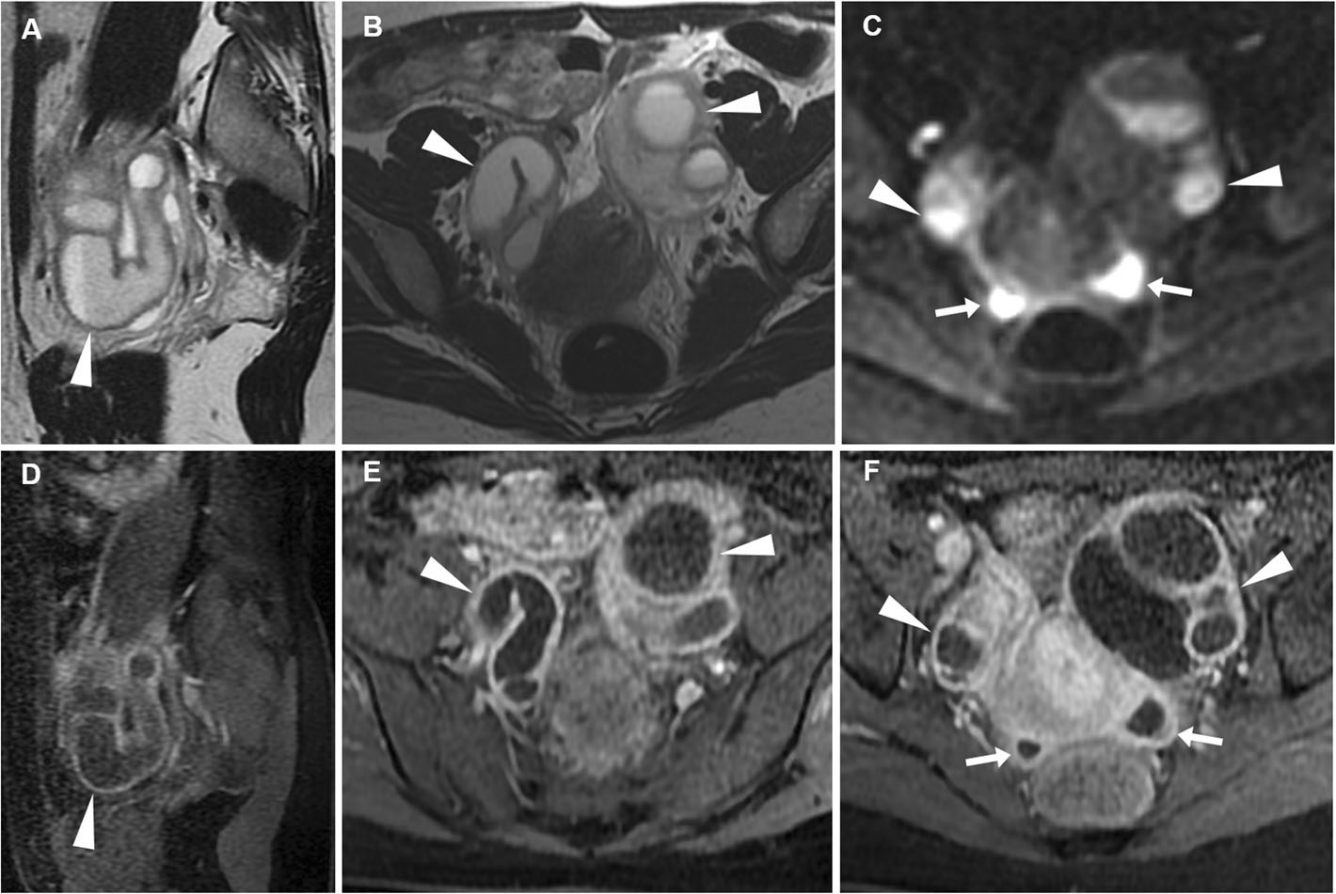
Acute pyosalpinx in the same patient as Fig. 11. Sagittal (a) and oblique-axial T2-weighted (b) images show distended fallopian tubes (arrowheads) with thickened walls and internal fluid-fluid levels. Axial DWI (*b* = 800 s/mm^2^) image (c) demonstrates high signal intensity indicating restricted diffusion of the tubal content (arrowheads) and of two fluid collections in the Douglas pouch (arrows), reflecting purulent content. Sagittal (d) and oblique-axial gadolinium-enhanced fat-suppressed (e, f) T1-weighted images show intense mural enhancement of the involved Fallopian tube (arrowheads) and of the fluid collections (arrows)
